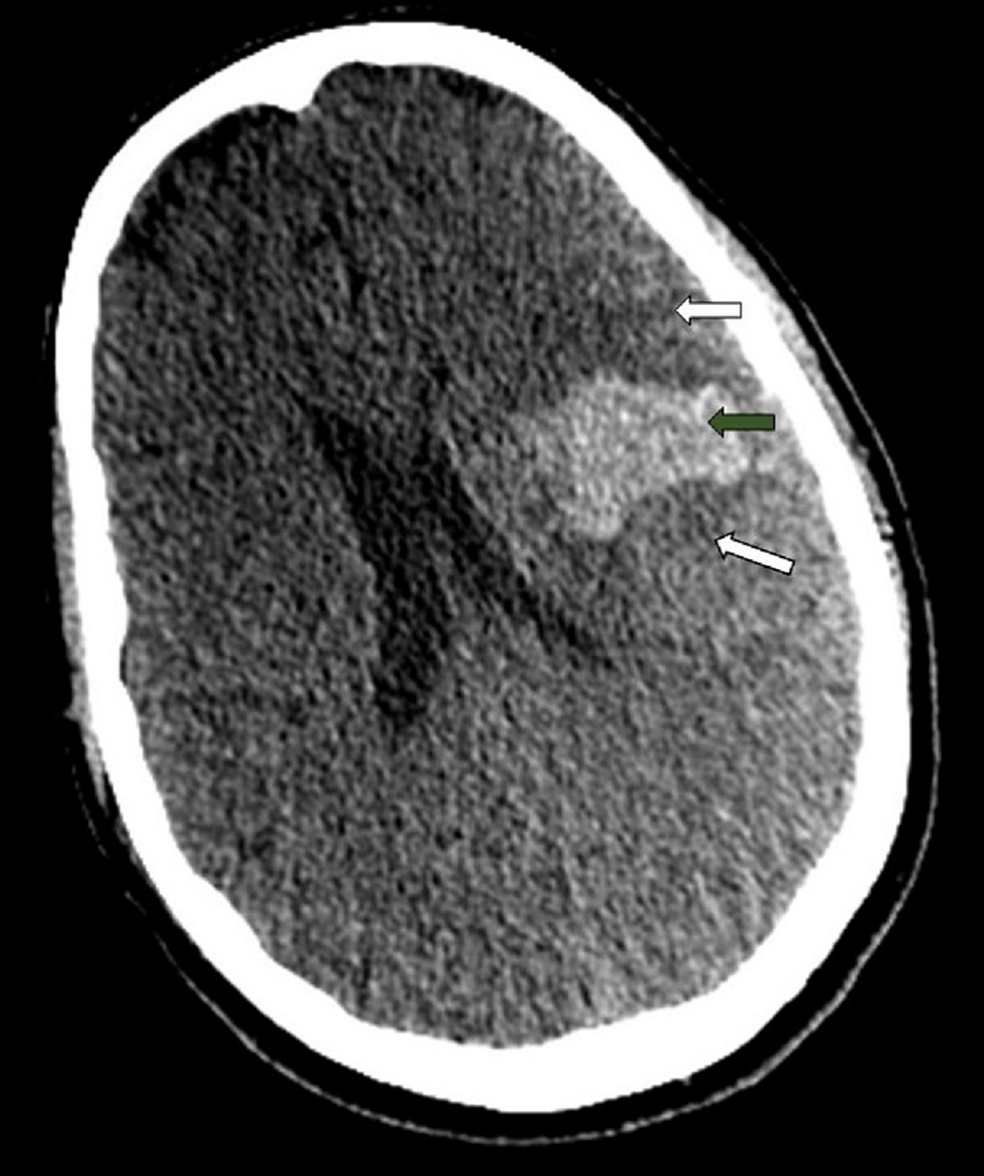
Cerebral CT, axial slice, without injection of contrast. Left deep parietal hematoma (green arrow), with perilesional edema (white arrow) and right falcique engagement. CT: computed tomography
